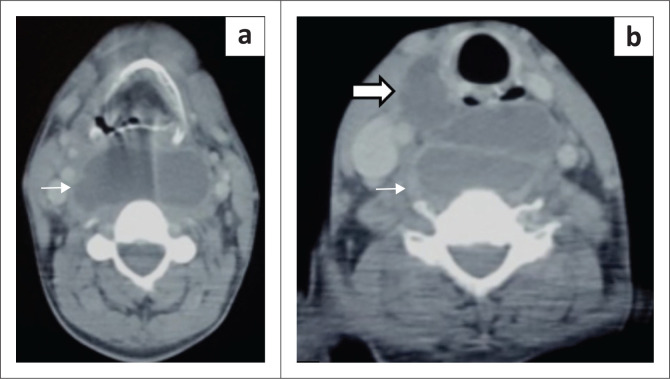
Axial contrast-enhanced CT scan of the neck, at the level of the hyoid bone (a) and at the level of sixth cervical vertebra (b), reveals a peripherally enhancing cystic lesion (thin arrows in a and b) involving the retropharyngeal and prevertebral space with extension to the anterior cervical and visceral space (thick arrow in b).
